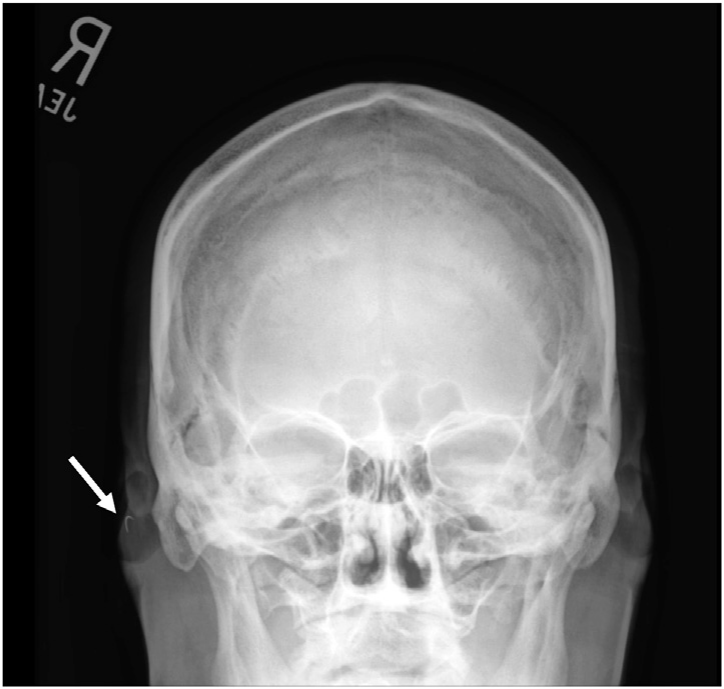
Skull x-ray indicating retained suture needle (*white arrow*).
